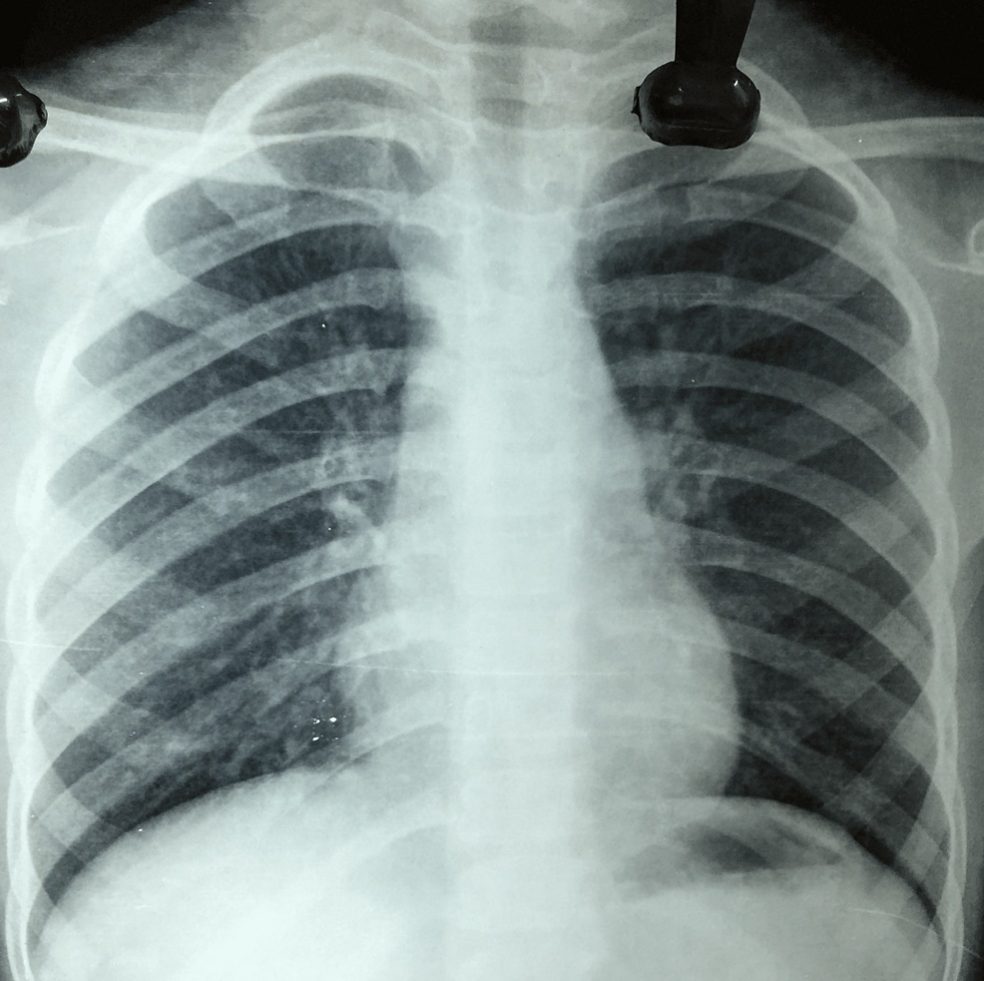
Chest radiograph PA view PA - posteroanterior
